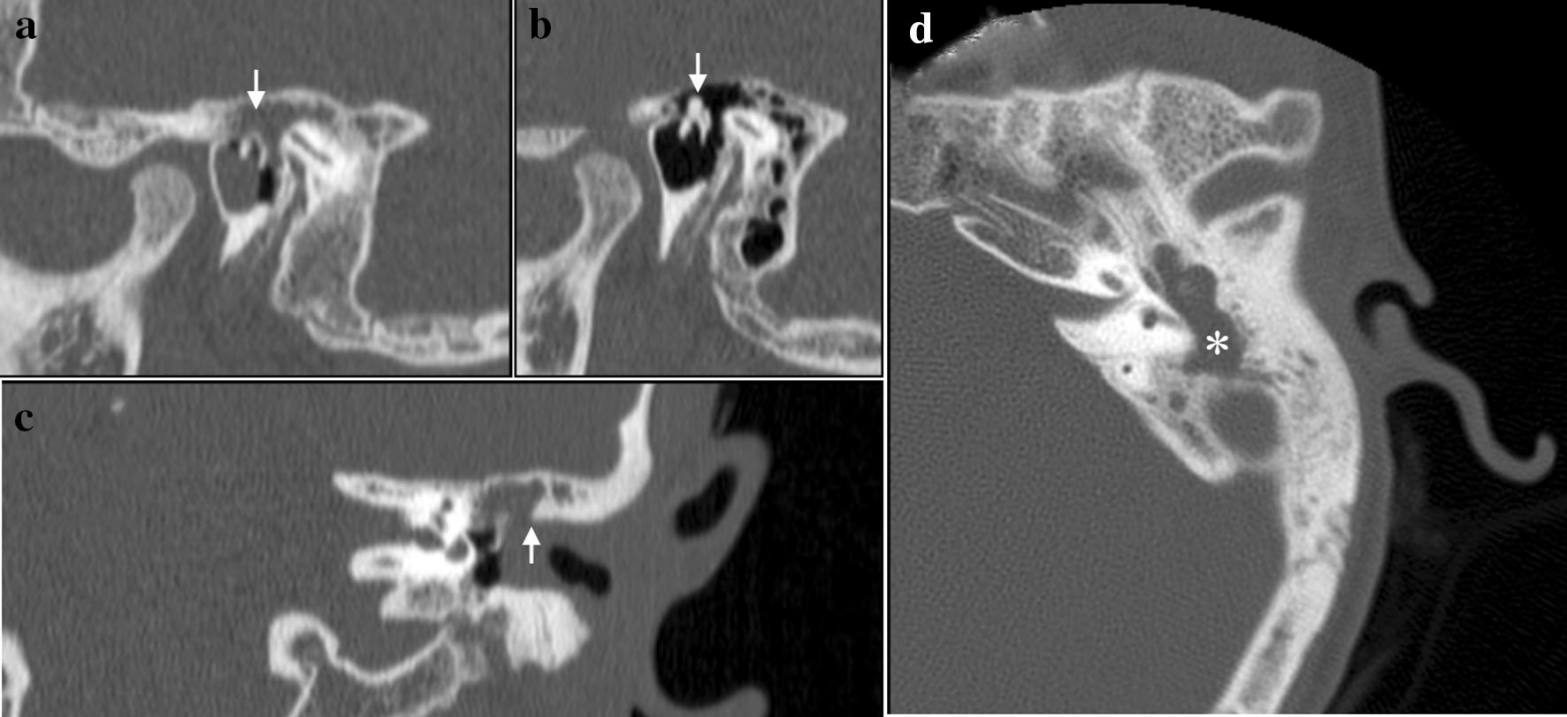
Cholesteatoma of the left middle ear in a 14-year-old boy in high-resolution CT. a Osteolysis of the head of the malleus and the body of the incus (*arrow*) in the sagittal plane (multiplanar reconstruction). Epitympanum is filled by soft-tissue mass (cholesteatoma). b Normal ear in the sagittal plane for comparison. c Destruction of scutum, again soft-tissue mass is visible in epitympanum (coronal plane). The ossicles are typically displaced medially. d Enlargement of the tympanic antrum with a soft-tissue mass within (*asterisk*)
